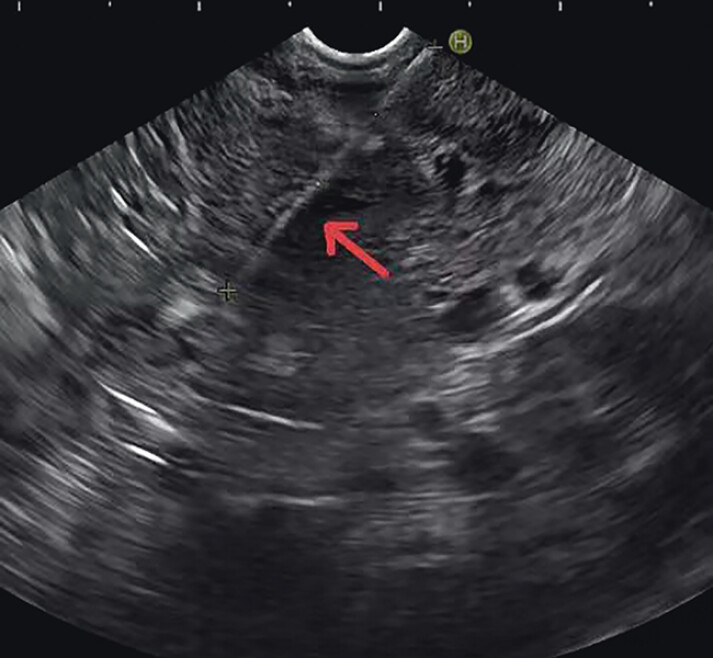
Endoscopic ultrasound image showing a strong echo signal in the eminence of the stomach, with one end located superficially in the submucosa and the other end penetrating the stomach wall and inserting into the pancreas.
